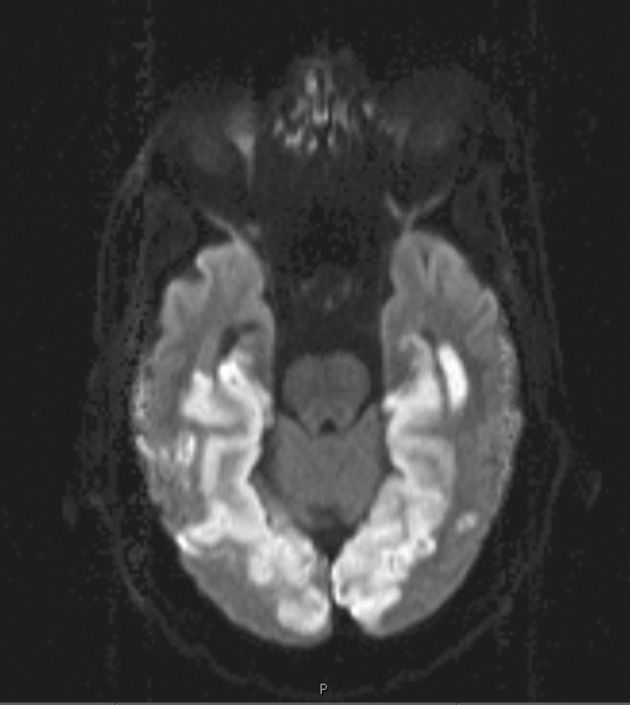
MRI Brain, diffusion‐weighted imaging. These representative axial MRI diffusion‐weighted images reveal areas of diffusion restriction in the bilateral PCA distributions (medial temporal lobes, occipital lobes).
